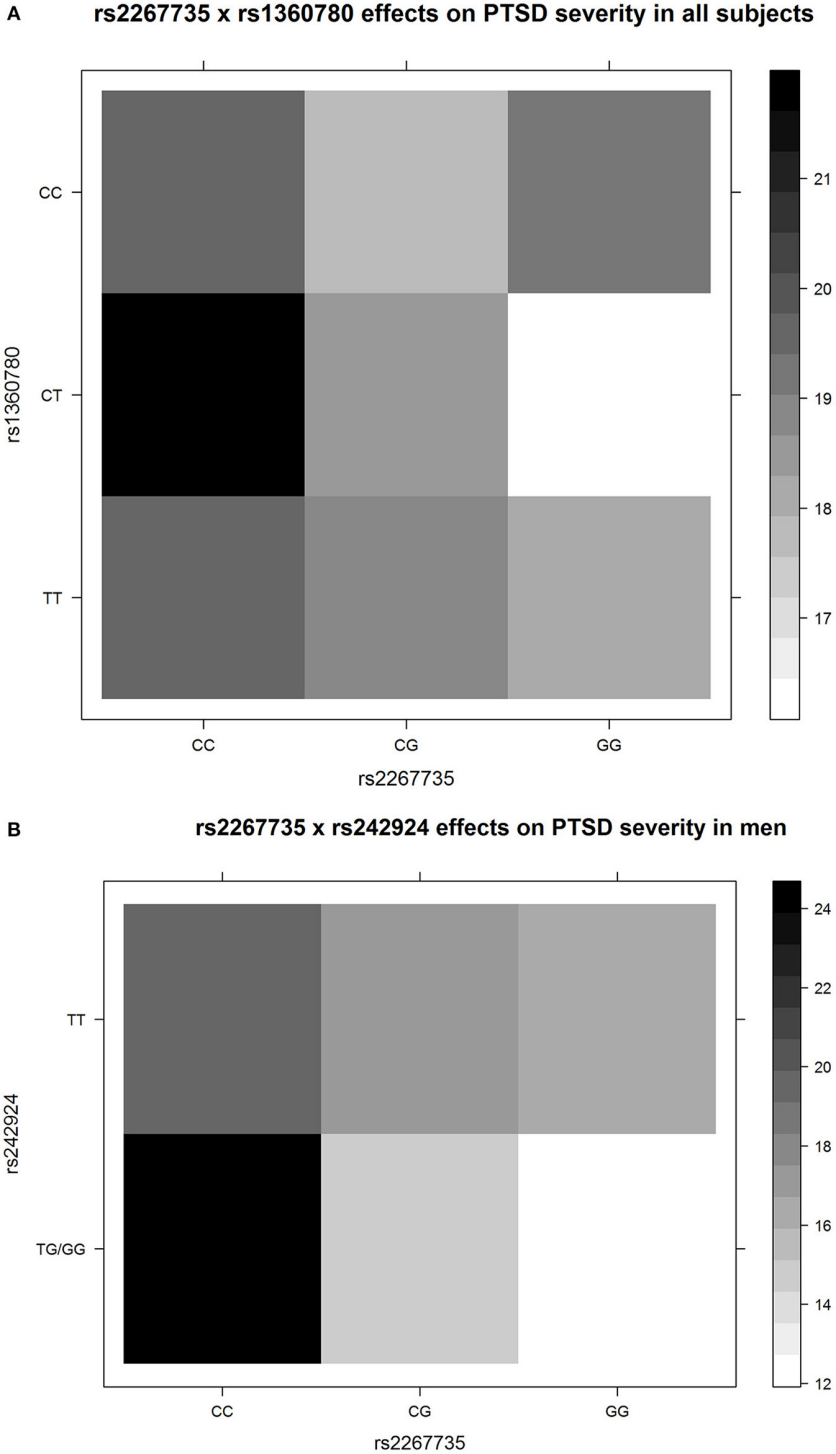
The G × G (epistasis) effects represented by the distributions of posttraumatic stress disorder (PTSD) severity across genotype combinations. The colors of each unit were filled according to the means of PTSD severity of the subjects with the corresponding specific genotype combinations. The upper panel (A) shows rs2267735 × rs1360780 for all subjects, and the lower panel (B) shows rs2267735 × rs242924 for men. Only three men had the rs242924 genotype GG; thus, we merged GG and TG. The figures directly show epistasis. For example, in (A), for the subjects with rs1360780 genotype CC, the minor allele G of rs2267735 is not correlated to PTSD severity; for the subjects with rs1360780 genotypes CT and TT, the minor allele G of rs2267735 corresponds to decreased PTSD severity, with different effect sizes. The figures were drawn by R3.4.4.
